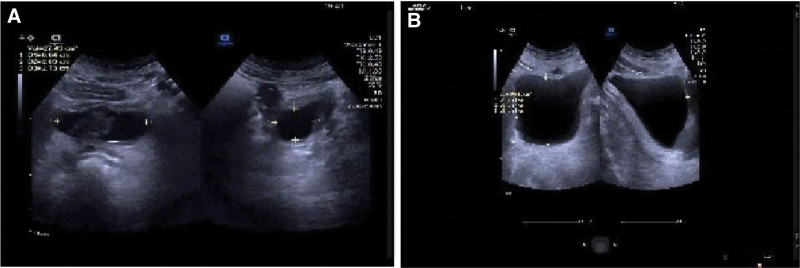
A. On September 22, 2022, a follow-up ultrasound of bladder residual urine showed 27 mL of residual urine in the bladder after urination. B. Urinary retention developed again after catheter removal, and the performed ultrasound showed a residual urine volume of 166 mL.
